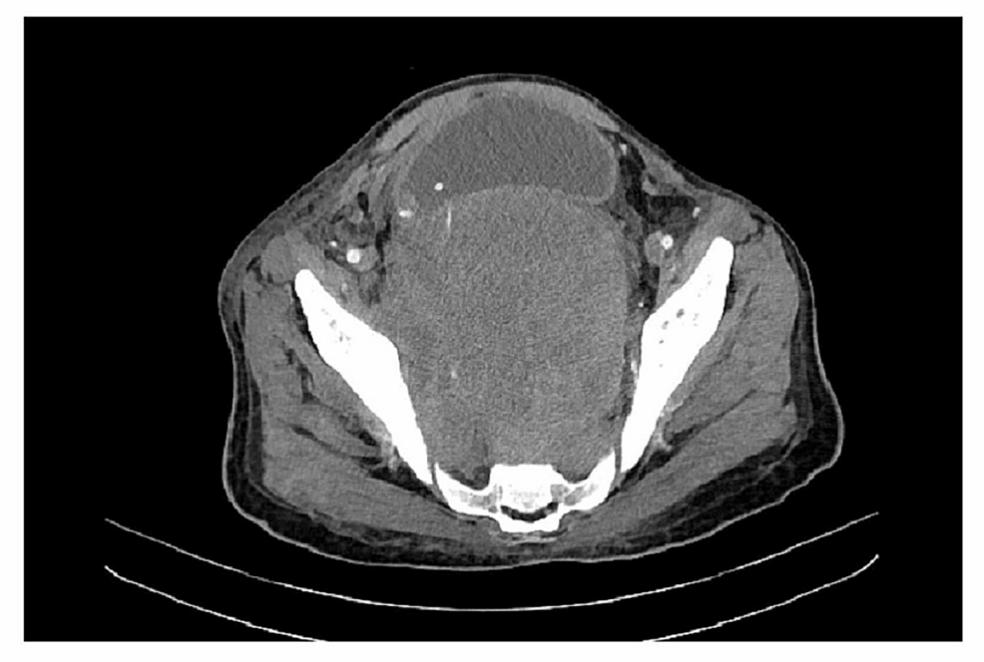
Pelvic CT scan: a retroperitoneal mass measuring 12.5 cm occupying the pelvic cavity. CT, Computed tomography.
